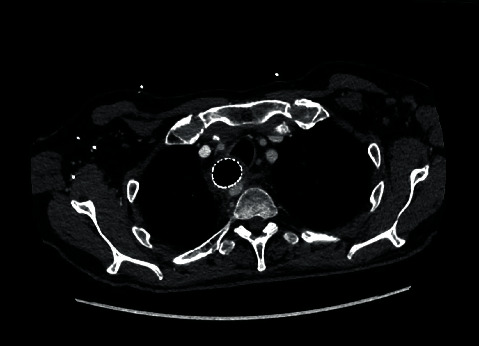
Axial CT angiogram slice demonstrating ARSA crossing behind the oesophagus with oesophageal stent in situ.
